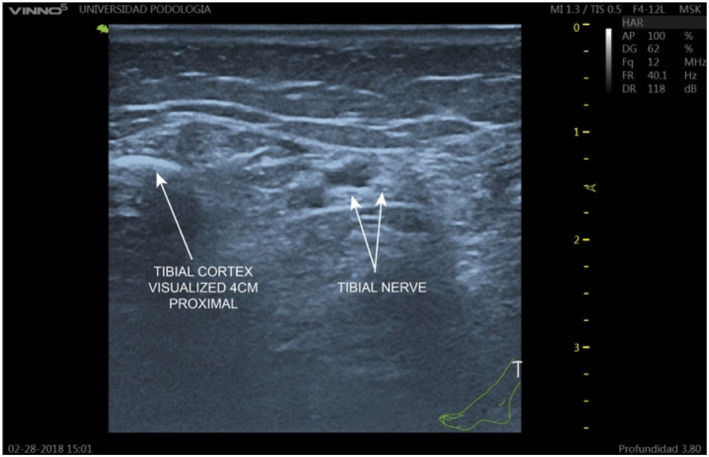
Ultrasound image of the tibial nerve (TN) at the supramalleolar level. The tibial nerve is identified as a predominantly hyperechoic structure with internal hypoechoic fascicles, displaying a characteristic honeycomb appearance, adjacent to the posterior tibial artery (PTA), which appears as a round anechoic structure. The tibial cortex, visualized 4 cm proximal to the inferior border of the medial malleolus, is seen as a curvilinear hyperechoic line, with its medial margin corresponding to the free cortical edge.
